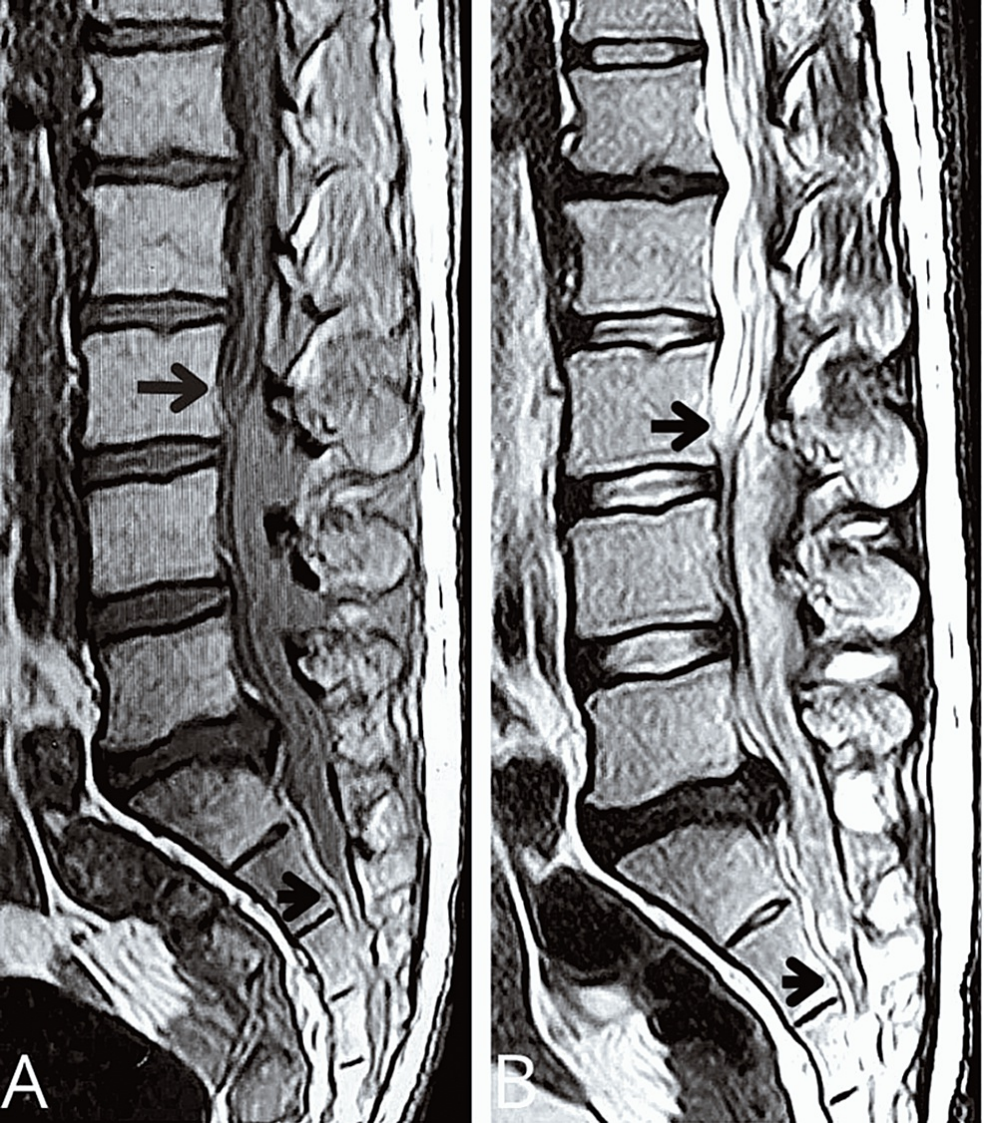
(A) Isointense lesion extending between L3-S2 segments in T1 MRI and (B) hyperintense lesion extending between L3-S2 segments in T2 MRI.
